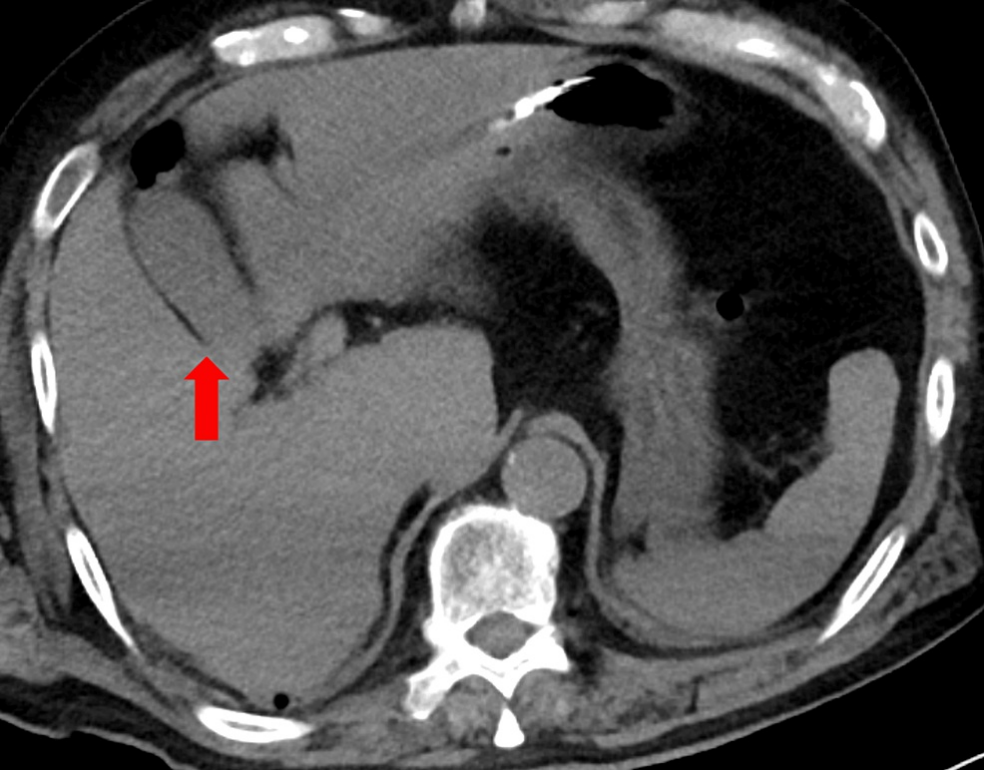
Computed tomography of the gallbladder upon admission There is no gallbladder swelling or bile mud (arrow).
